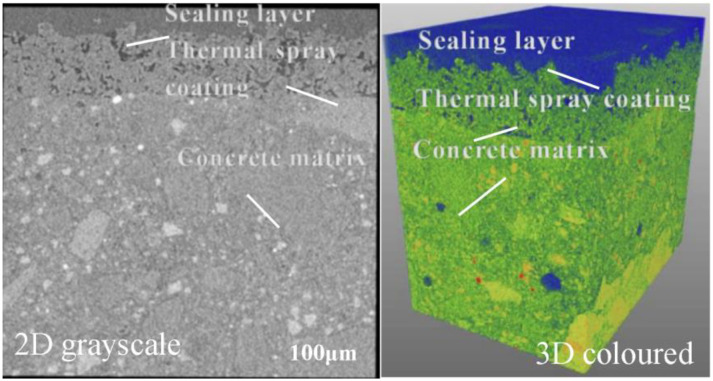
CX-CT 3D reconstruction.
